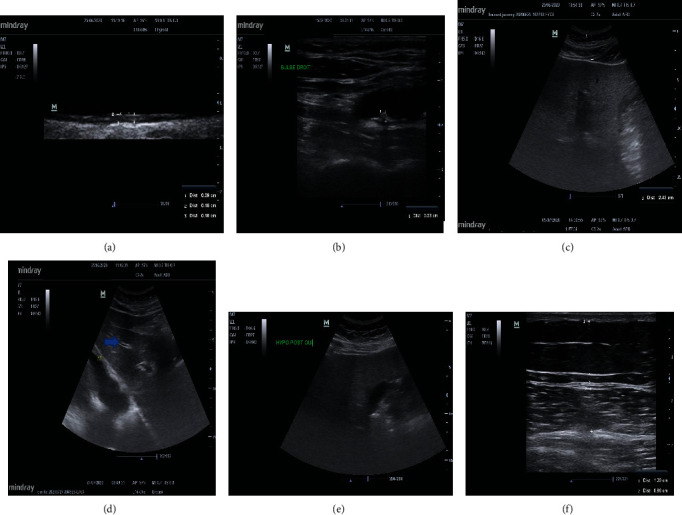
(a) Normal carotid artery ultrasonography. High-frequency probe. The intima media thickness is measured at three points. (b) Visualization of a plaque of 0.23 mm thickness located near the carotid bifurcation. High-frequency probe. (c) Liver echography with determination of the skin capsular distance (2.43 cm). Low-frequency curvilinear probe. (d) Normal liver echography showing the portal veins (horizontal arrow), the diaphragm, and a homogeneous parenchyma. Low-frequency curvilinear probe. (e) Nonalcoholic steatohepatitis. This picture shows a rapid ultrasound attenuation. The vessels are attenuated, and the posterior liver is not seen. (f) Measurement of the rectus femoris (1) and vastus intermedius (2) thickness. High-frequency linear probe.
